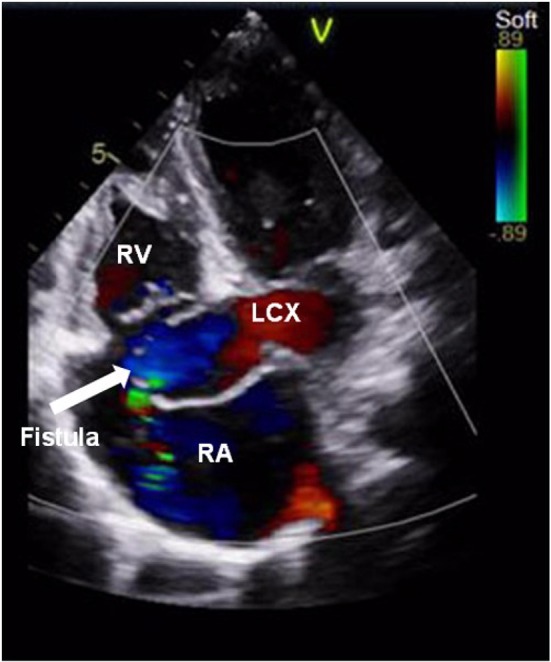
Color Doppler examination showed a coronary artery fistula from the left circumflex artery to the right atrium. The arrow points to the fistula. RV, right ventricle; LCX, left circumflex artery; RA, right atrium.
